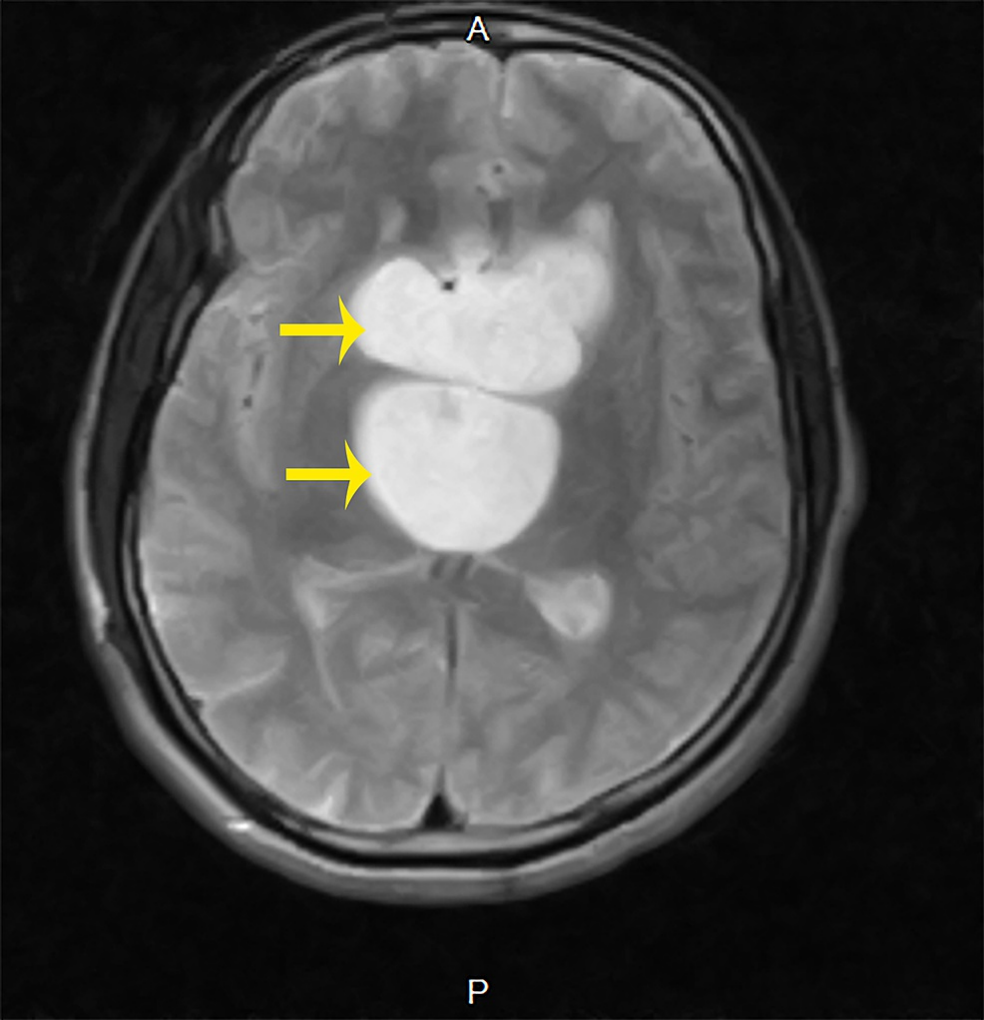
T2-weighted brain MRI showing contrast-enhancing space occupying the lesion in the suprasellar compartment.
